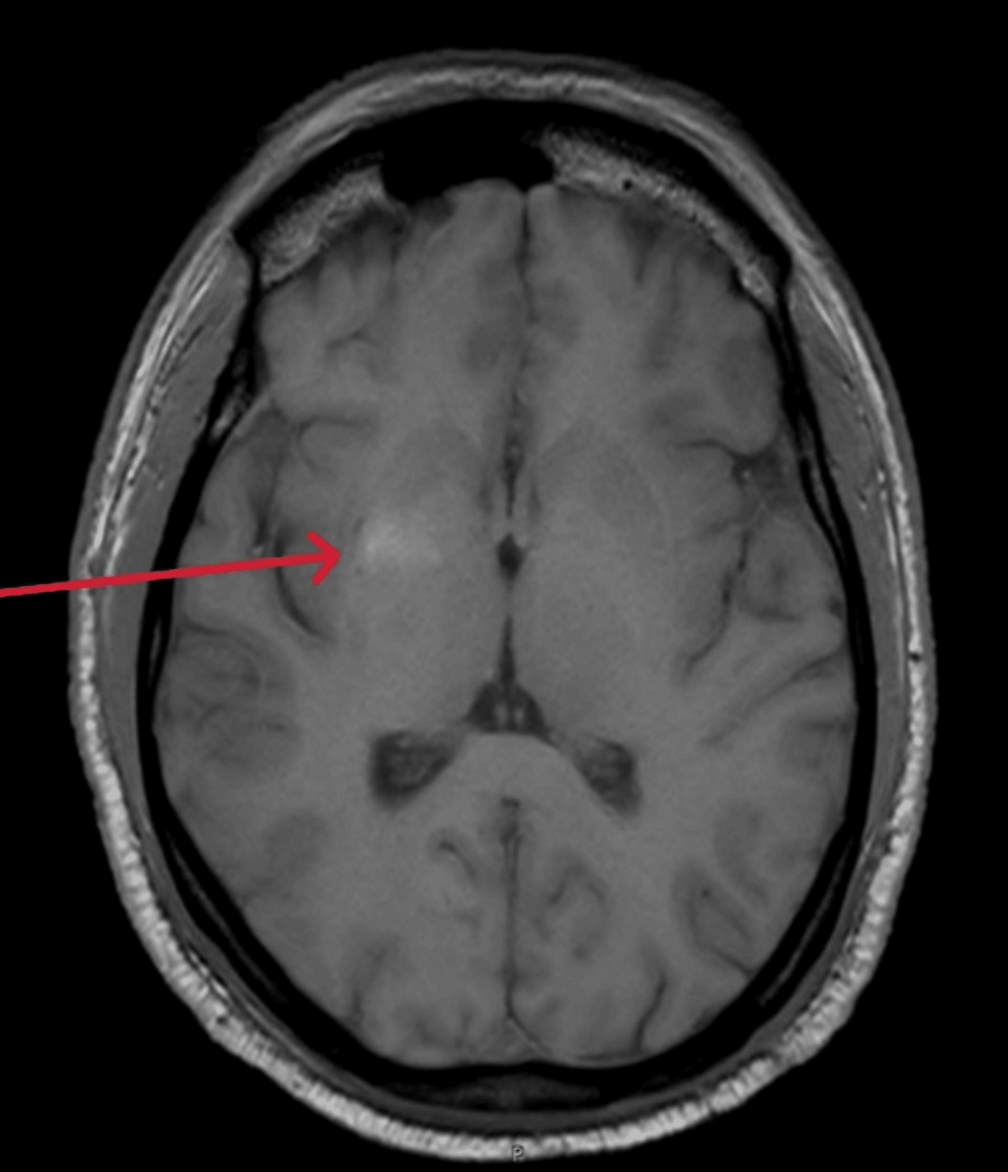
T1-weighted magnetic resonance imaging (axial view) showing hyperintensity noted in the right basal ganglia (red arrow)
